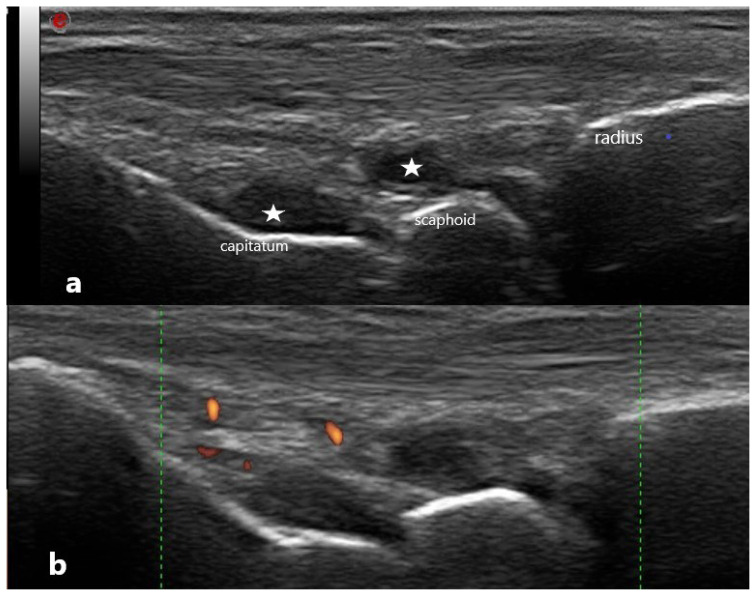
US image of the wrist of a 36-year-old patient with a 5-year diagnosis of RA, with no joint sensitivity or swelling, and using methotrexate 20 mg/week and hydroxychloroquine 200 mg/day. Sedimentation rate is 2 mm/s, CRP is 0.1 mg/dL, DAS is 28, ESR is 0.49, SDAI is 2, and CDAI is 2. Gray scale (a) shows fluid in the joint space, and there is a weak Doppler signal on the wrist (b). This patient is in remission clinically but not sonographically. The stars indicate synovial fluid.
